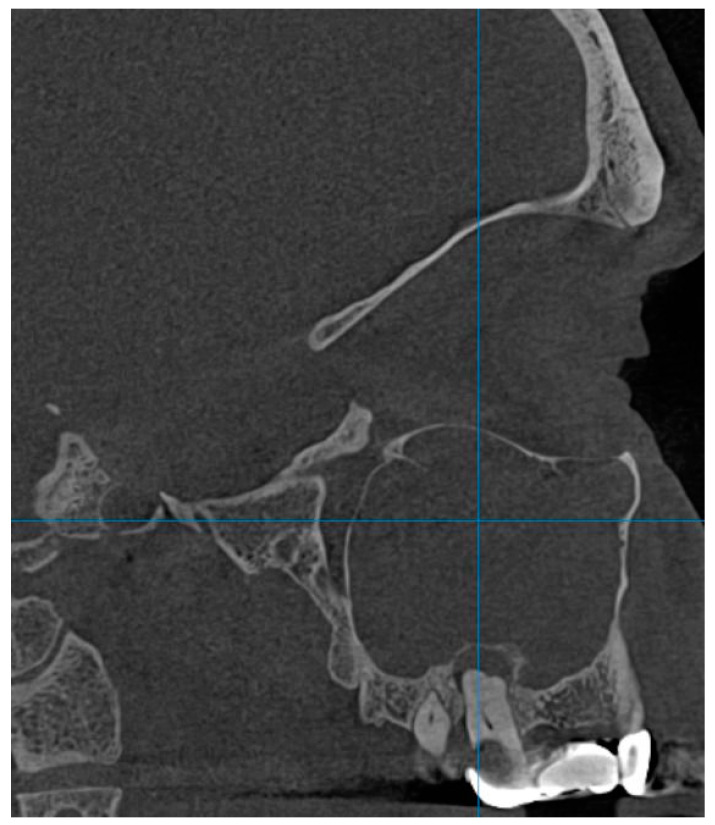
Completely opacified left maxillary sinus following chronic maxillary sinusitis consecutive to chronic periapical osteitis 2.7.
